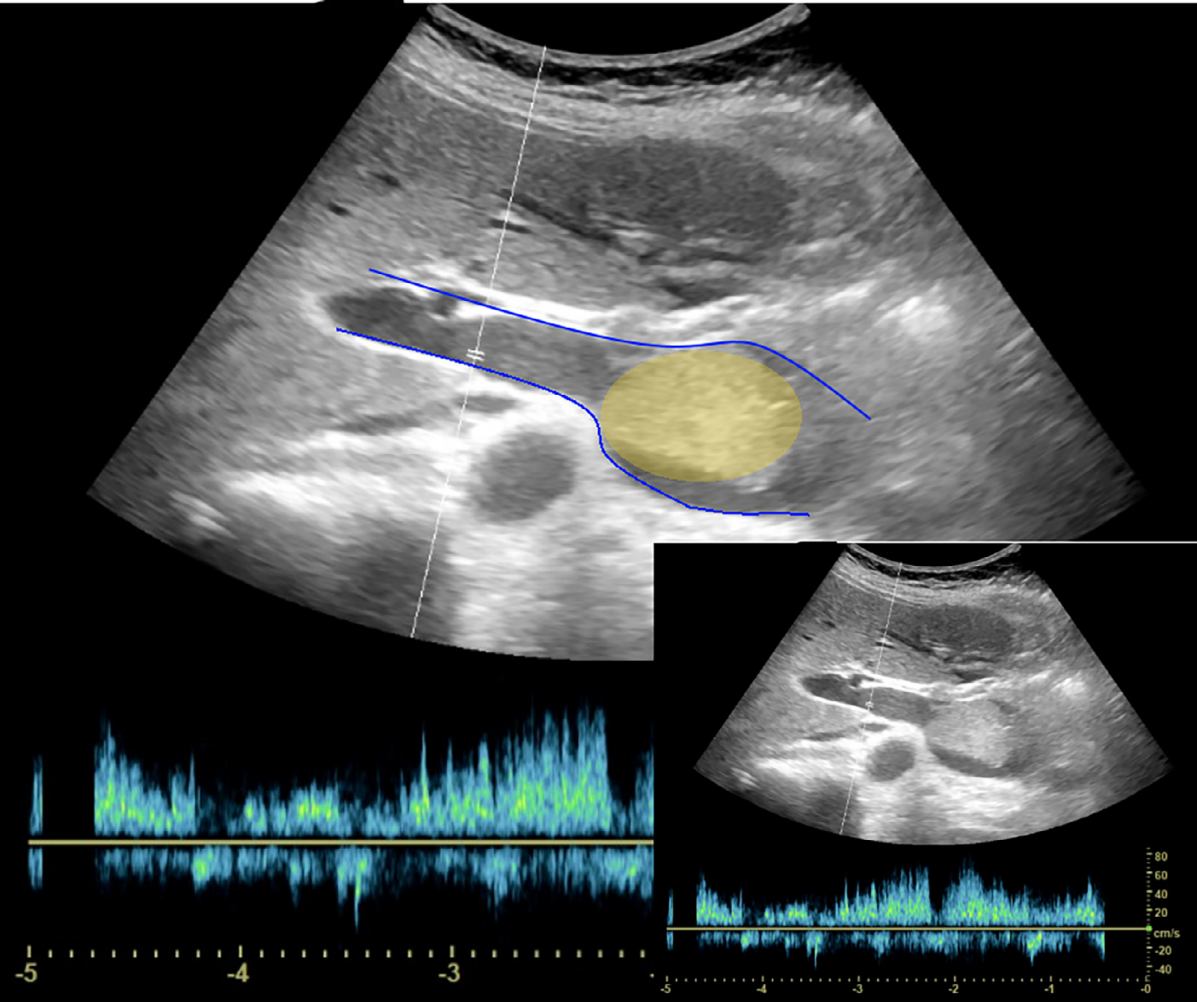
Ultrasound picture showing the thrombosis (yellow) inside the portal vein (blue).
